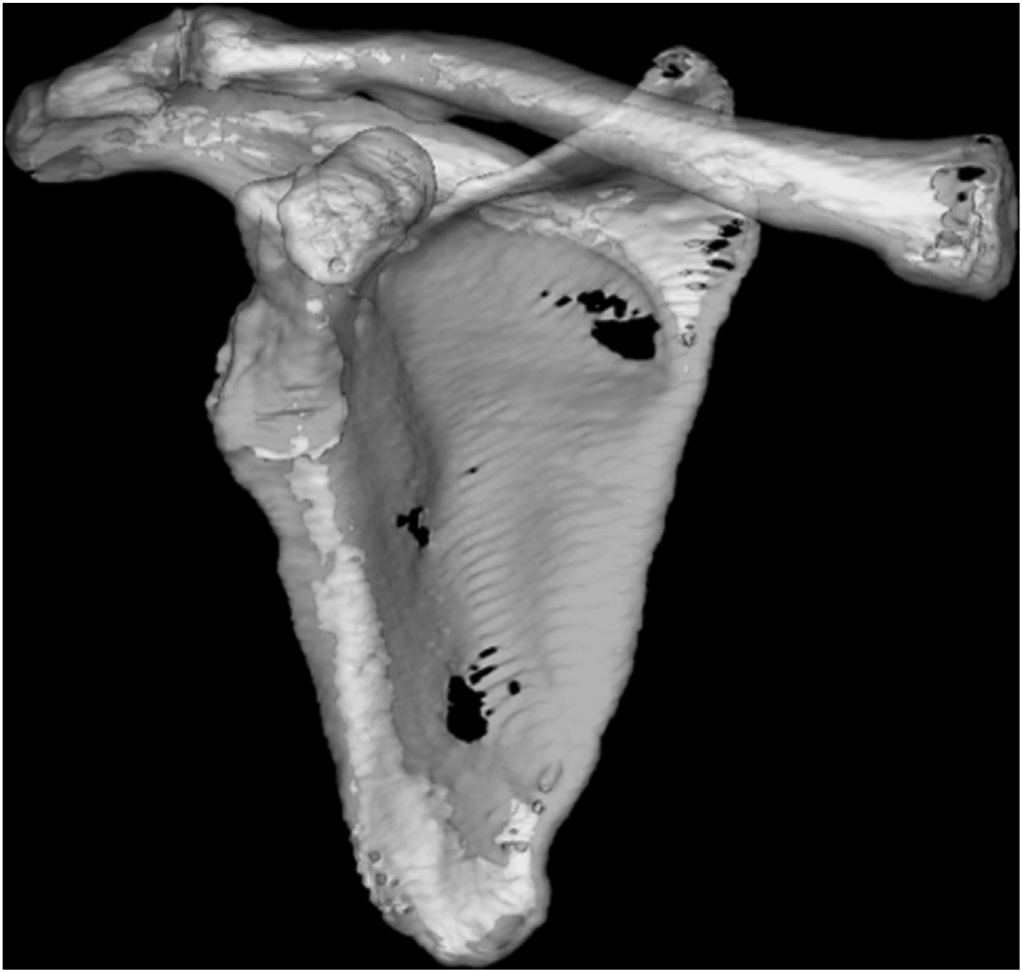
3-dimensional bone model created from preoperative computed tomography (CT) data of scapula.
